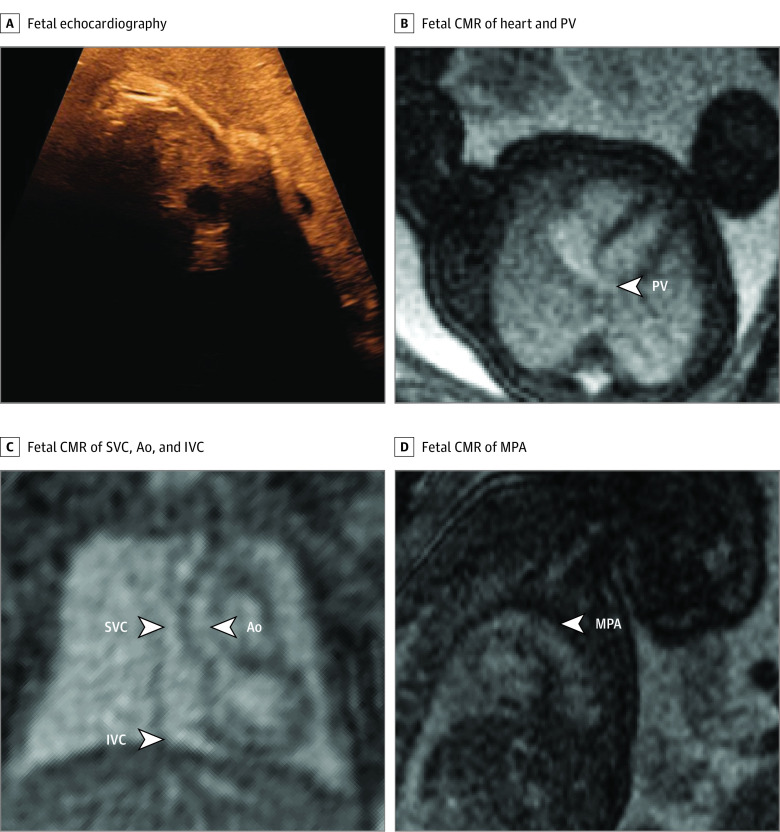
Basic Assessment in a Fetus With Risk Factors for Cardiac Malformation and a Very Poor Acoustic Window The fetal heart and vessels were not visible at all during fetal echocardiography, due to mother having obesity (A). Fetal cardiovascular magnetic resonance imaging (MRI) showed normal-sized ventricles with normal systolic function and at least 1 normal pulmonary vein (PV) without obvious direct or indirect signs of aberrant pulmonary veins (B), superior venae cavae (SVC) and inferior venae cavae (IVC) and normal ascending aorta (Ao) (C), and normal main pulmonary artery (MPA) (D). This information ruled out major congenital heart disease, and delivery was planned at the hospital closest to the patient’s home. A nonurgent postnatal echocardiography examination was performed before discharge, which showed a normal cardiovascular anatomy. Contrast and brightness in the CMR images have been optimized for printing (case 30) (eTable in the Supplement).
